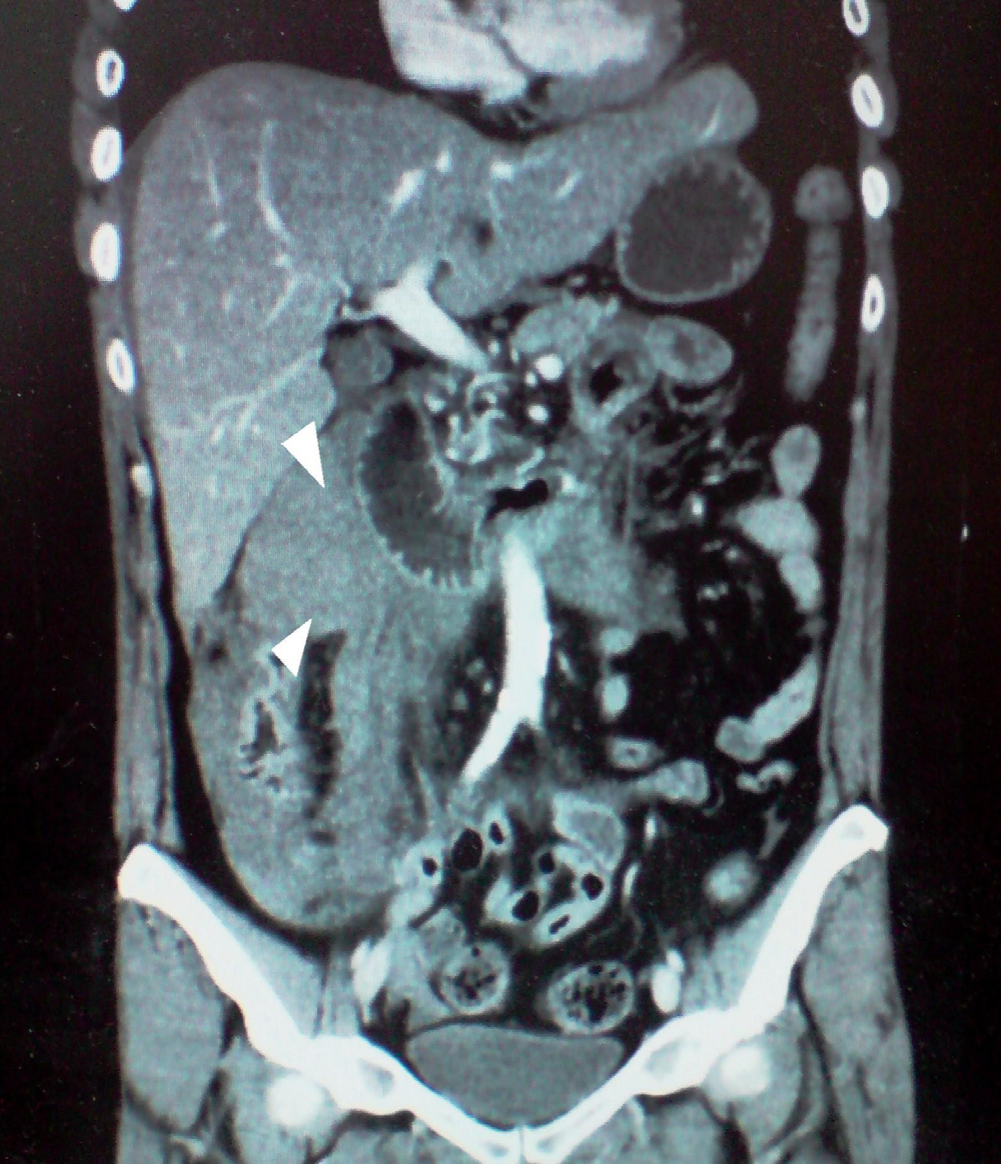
Paraduodenal hematoma shown in the coronal Abdominal CT with contrast. (White arrowhead).
